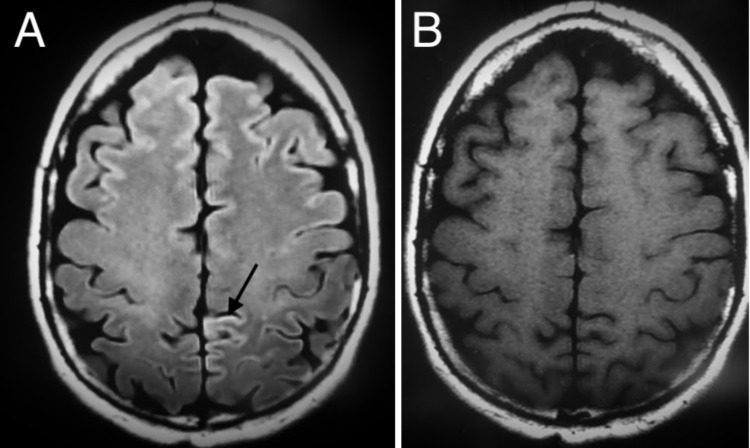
MR image of the brain with and without contrast (A) T2 FLAIR sequence transverse view shows an area of signal intensity along the paramedian portion of the left parietal lobe consistent with cortical ribboning in comparison to (B) T1 sequence (arrow).
